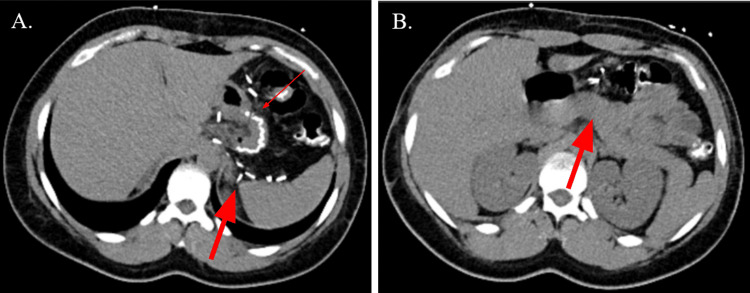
Non-contrast CT abdomen and pelvis (A) Axial view of the abdomen demonstrating several clips for vessel sealing (thick arrow) and the staple line (thin arrow). (B) Axial view of the abdomen at the level of the pancreas (thick arrow) without signs of acute inflammation.
